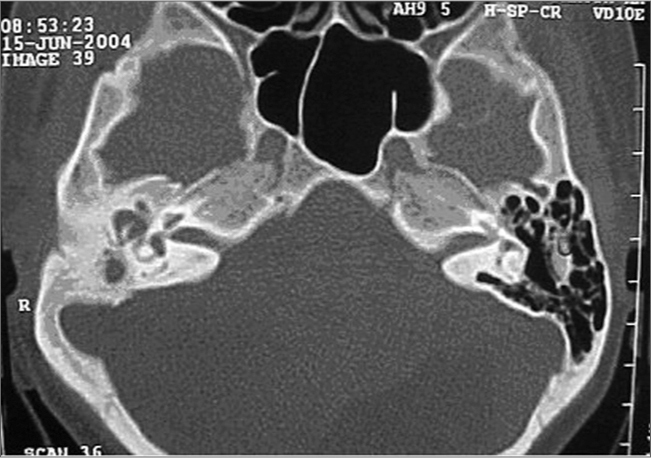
Middle ear papilloma - Post-op CT scan
